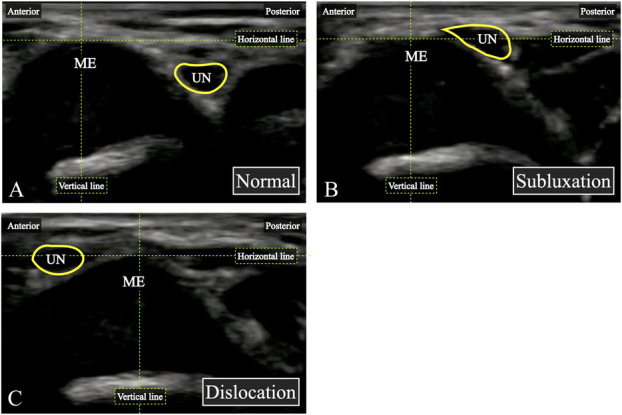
UN positional classification on short-axis ultrasound. The position of the UN was classified relative to 2 reference lines drawn from the apex of the ME. Fig. 2-A Type N (normal): The UN is located posterior to the vertical reference line and deep to the horizontal reference line within the cubital tunnel. Fig. 2-B Type S (subluxation): The UN is located posterior to the vertical reference line but superficial to the horizontal reference line. Fig. 2-C Type D (dislocation): The UN is located anterior to the vertical reference line. ME = medial epicondyle, and UN = ulnar nerve.
